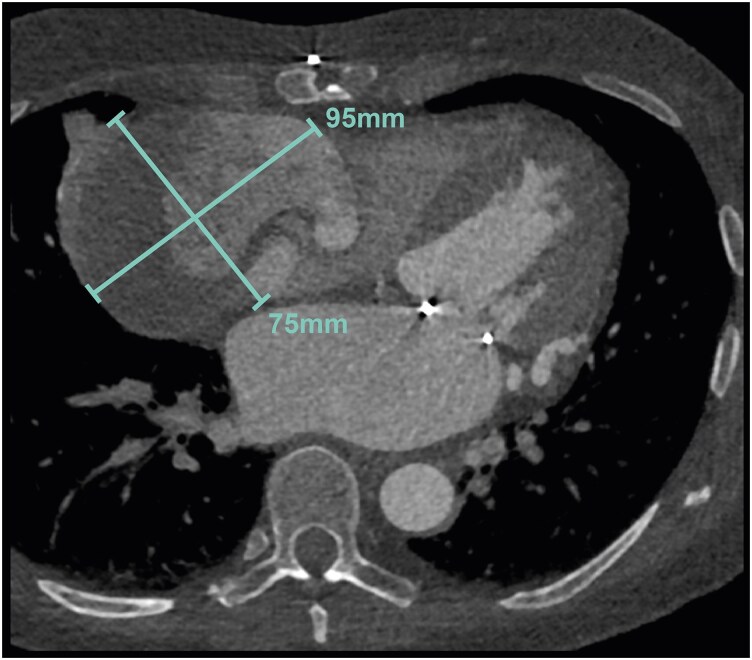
Cardiac computed tomography imaging of Patient 3 showing a dilated right coronary artery with a thrombosed 75 × 95 mm aneurysm.
